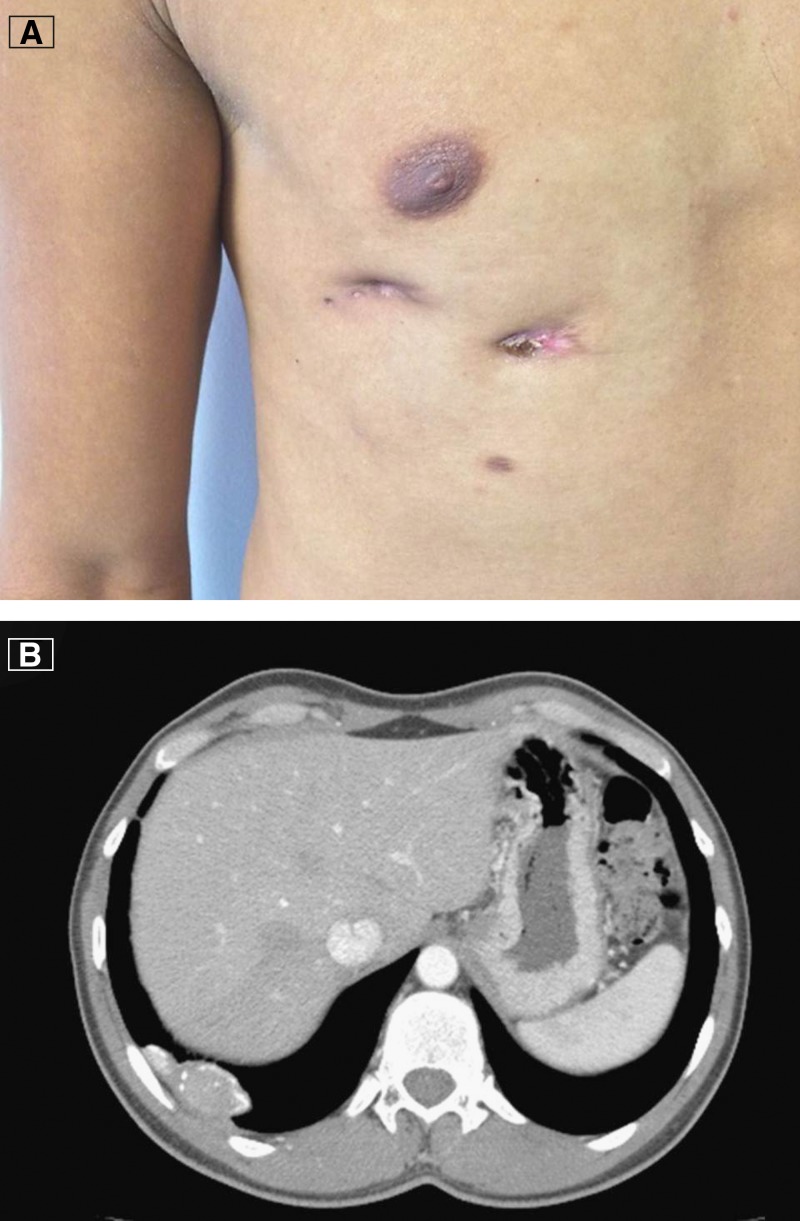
(A) Clinical picture of the case. (B) Thorax CT scan showing a 54 x 18 x 26-mm right lower lobe mass with peripheral calcifications.
